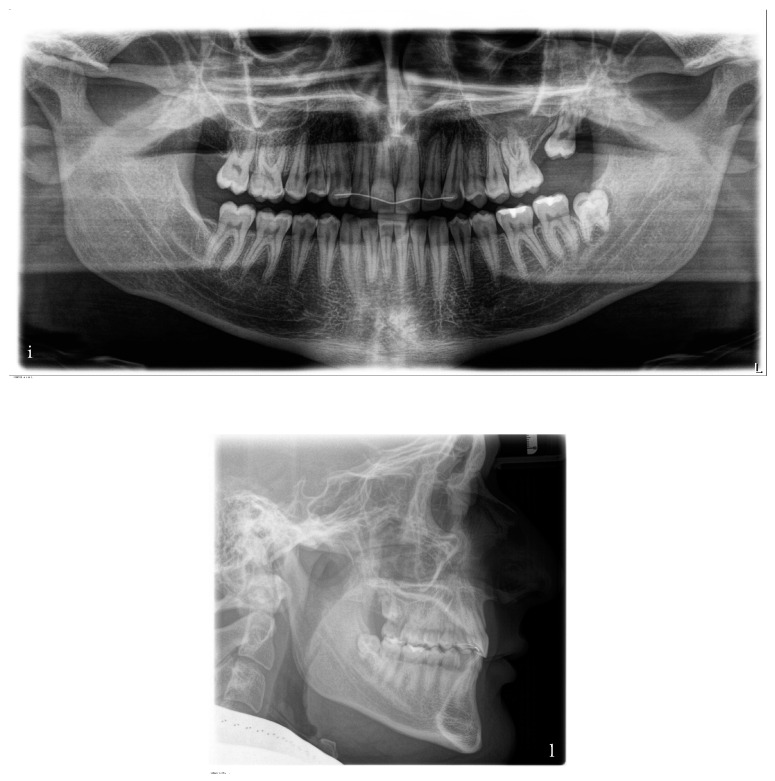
Final records of the patient after 28 months of treatment; (a–c) extraoral posttreatment pictures: lateral and frontal views; (d–f) intraoral posttreatment pictures: lateral and frontal views; (g,h) occlusal views: upper and lower arch; (i,l): posttreatment radiographs: panoramic and lateral cephalogram.
